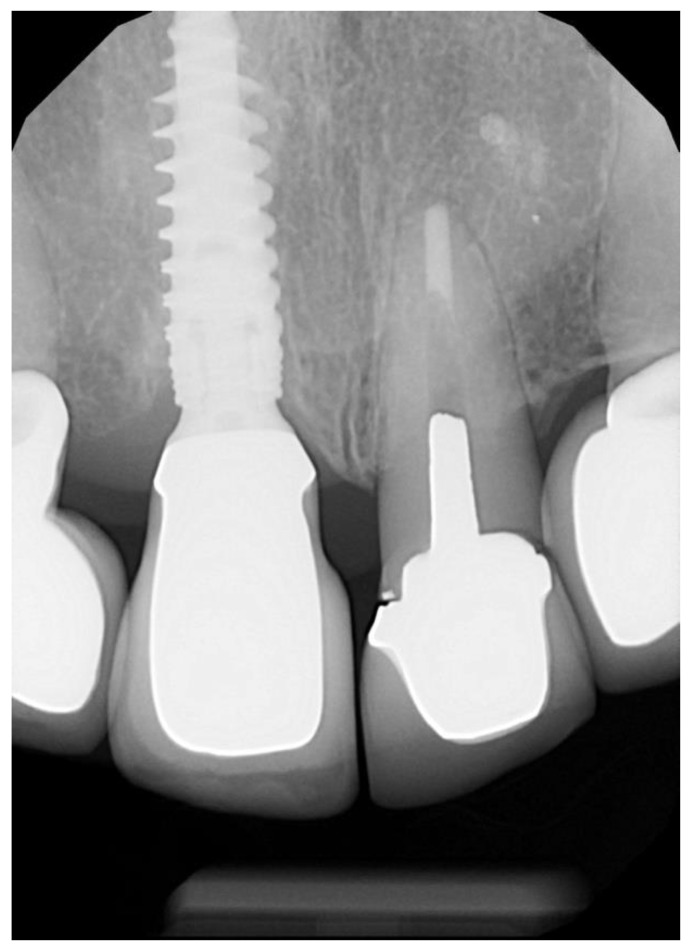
Pre-operative X-ray of 21 prior to shield preparation.
